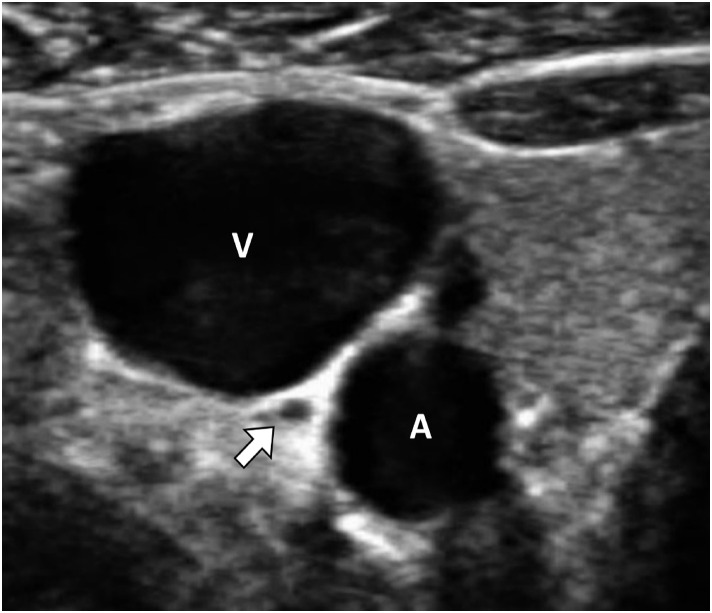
Transverse cross-sectional image of the vagus nerve by carotid ultrasound. The vagus nerve is shown as a small, rounded, hypoechoic structure between the carotid artery and jugular vein. The cross-sectional area of the vagus nerve was measured by manual tracing along the medial side of the hyperechoic epineural rim. The arrow indicates the vagus nerve. A, common carotid artery; V, jugular vein.
